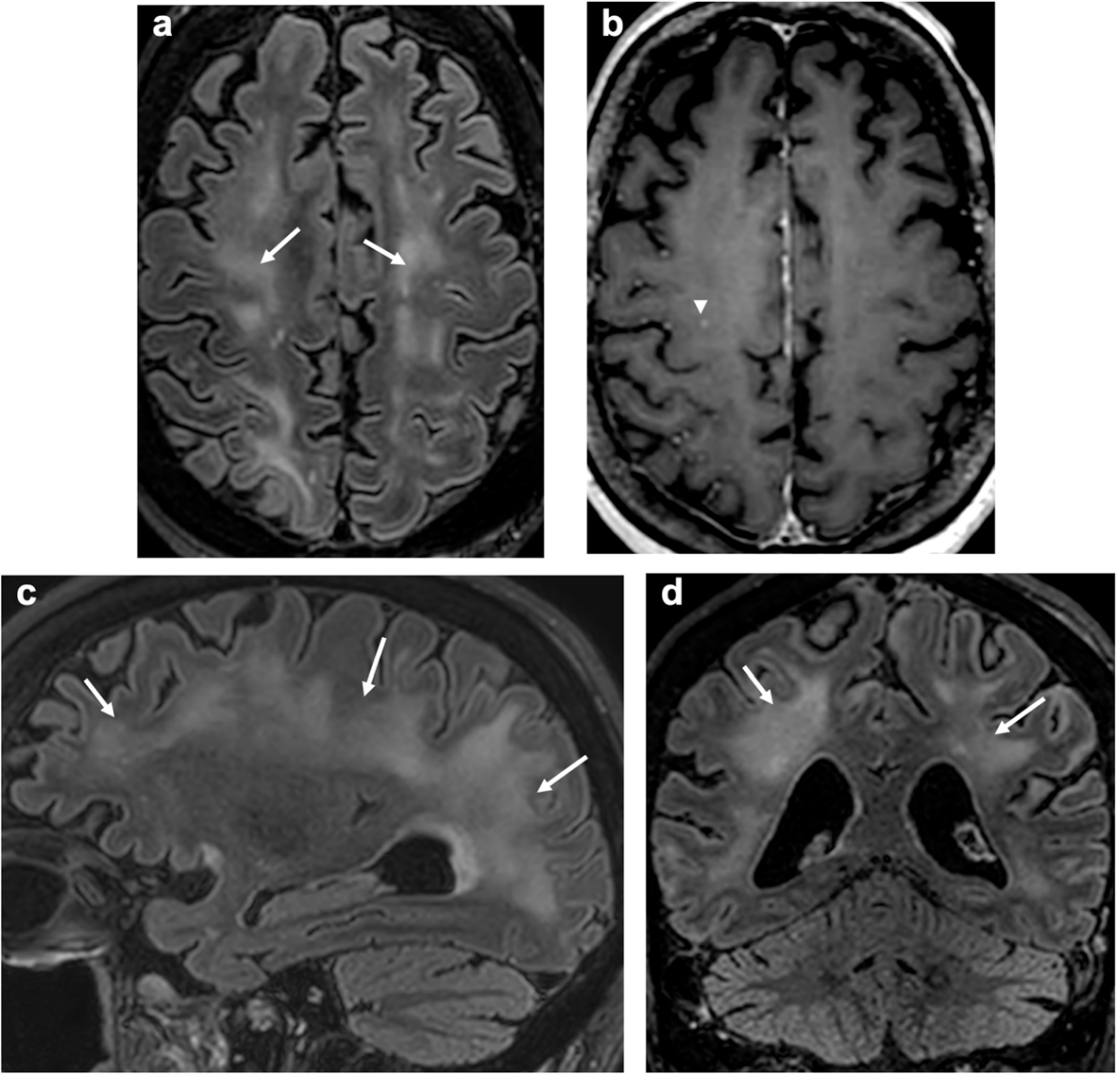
Axial, sagittal, and coronal FLAIR (a, c, d) and axial post-contrast T1 (b)-weighted MR images: extensive white matter confluent FLAIR hyperintensities (arrow), with small foci of contrast enhancement (arrow head)
